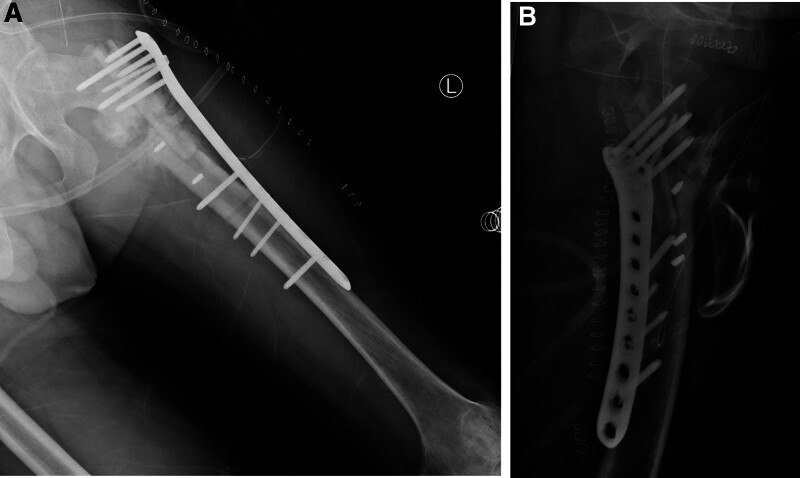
(A) Plain anteroposterior (AP) radiograph (B) lateral radiograph taken postoperatively after plate fixation with FA.
